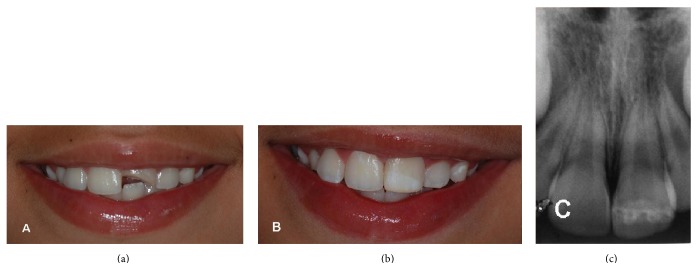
(a) Initial clinical aspect. (b) Clinical aspect shortly after bonding. (c) Final radiographic aspect.
